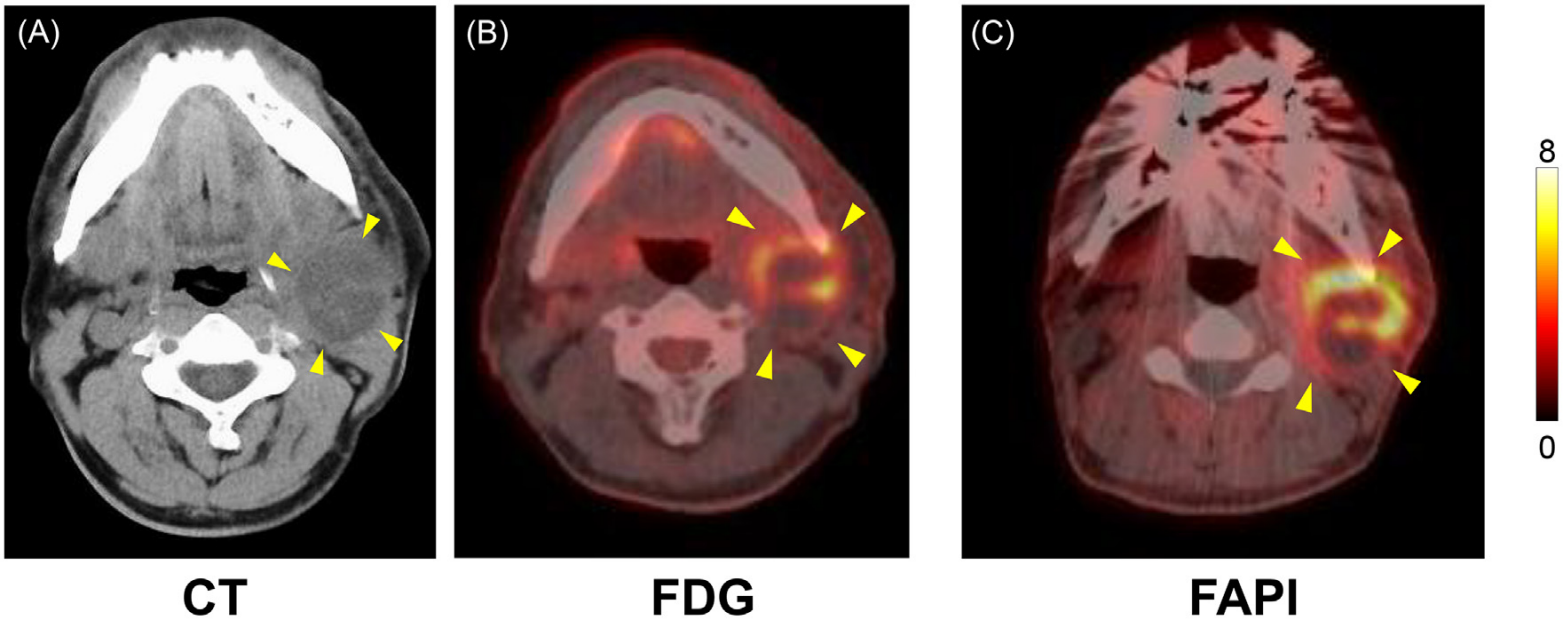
Multimodality imaging of the left level-II mass. (A) Axial noncontrast CT showing a 40 mm cystic–solid lesion with ill-defined margins (arrowheads). (B) Axial FDG PET/CT demonstrating intense tracer uptake (SUVmax 10.2) and (C) Axial ^18^F-FAPI-74 PET/CT at the identical slice level showing a similarly intense uptake (SUVmax 15.4). Color scale range 0-8, adjusted for visual comparison (actual SUVmax 15.4).
